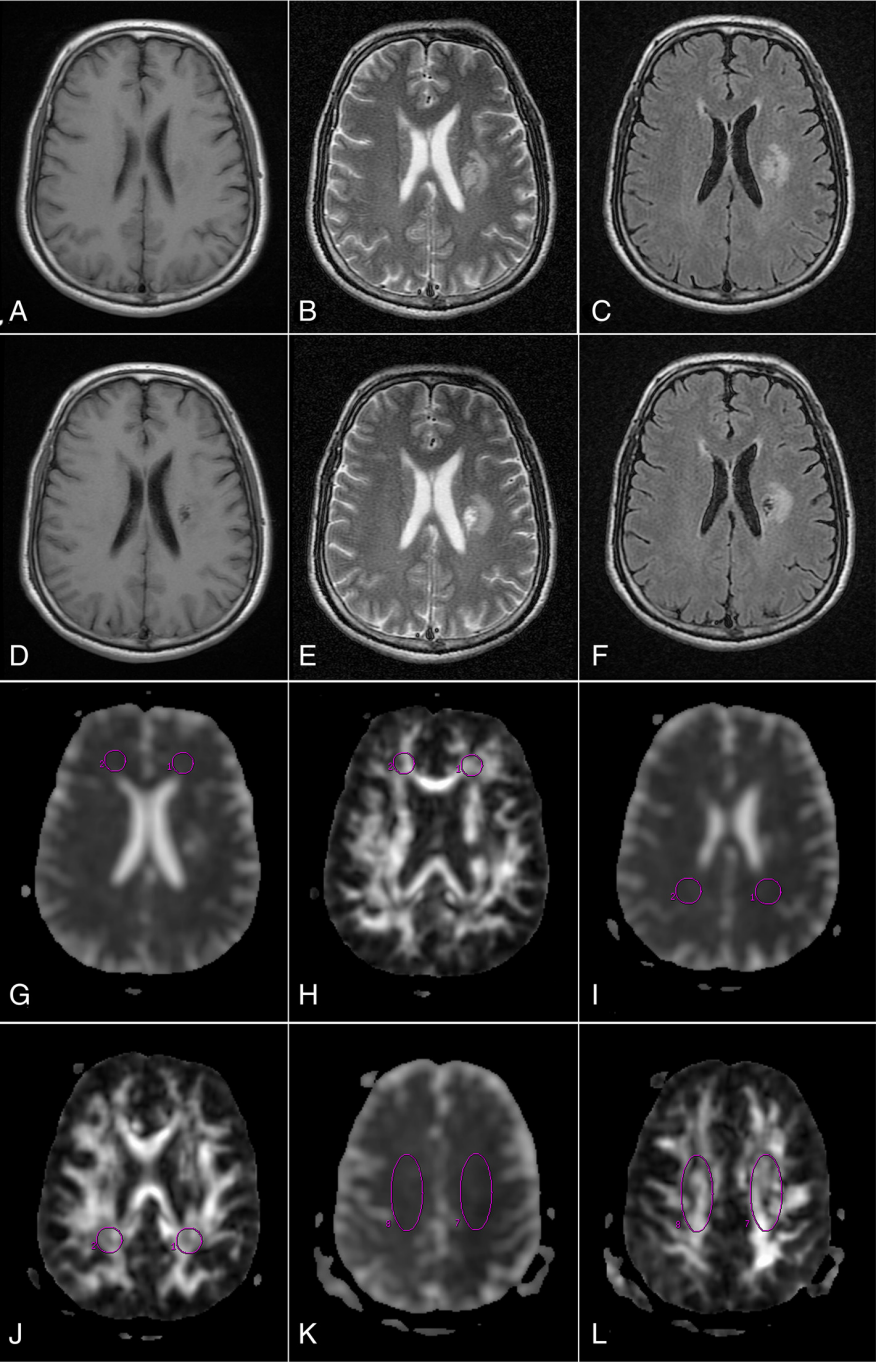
Ischemic lesion and regions of interest (ROIs) are shown in the traditional magnetic resonance and diffusion tensor magnetic resonance images from a patient with acute infarction at the left posterior corona radiata. (A) T1-FLAIR obtained at week 1 (W1) after stroke show a hypointense area at the left posterior corona radiata. (B) T2-weighted image and (C) T2-FLAIR images obtained at W1 show a hyperintense area at the left posterior corona radiata. (D), (E) and (F) show the lesion at W12. (G), (I) and (K) mean diffusivity images and (H), (J) and (L) fractional anisotropy images show ROIs in the left and right frontal white matter, posterior periventricular white matter and centrum semiovale.
